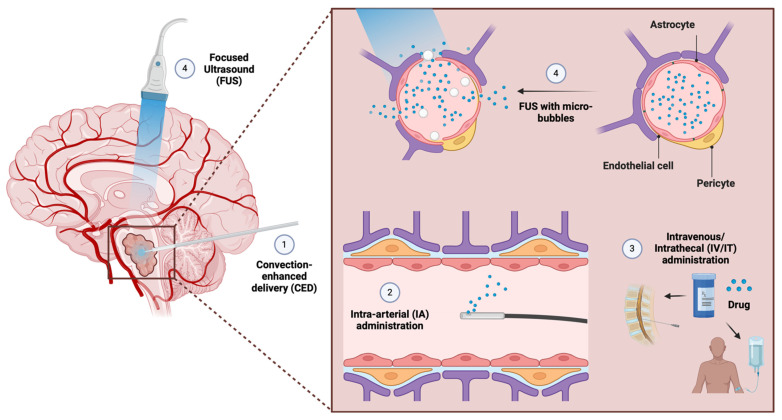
Methods of improving intratumoral drug delivery in DMG. 1. Convection-enhanced delivery (CED) bypasses the blood–brain barrier (BBB) to deliver drugs via micro-catheters and a positive pressure gradient. 2. Intra-arterial administration can directly deliver drugs to a target artery that feeds the tumor. Osmotic agents can be added to disrupt the blood–brain barrier. 3. IV/IT drug administration can overcome at least some first-pass metabolism and, for IT administration, bypass the BBB. 4. Focused ultrasound (FUS) can non-invasively and temporarily disrupt the blood–brain barrier; this technique can be combined with microbubbles for increased efficacy.
